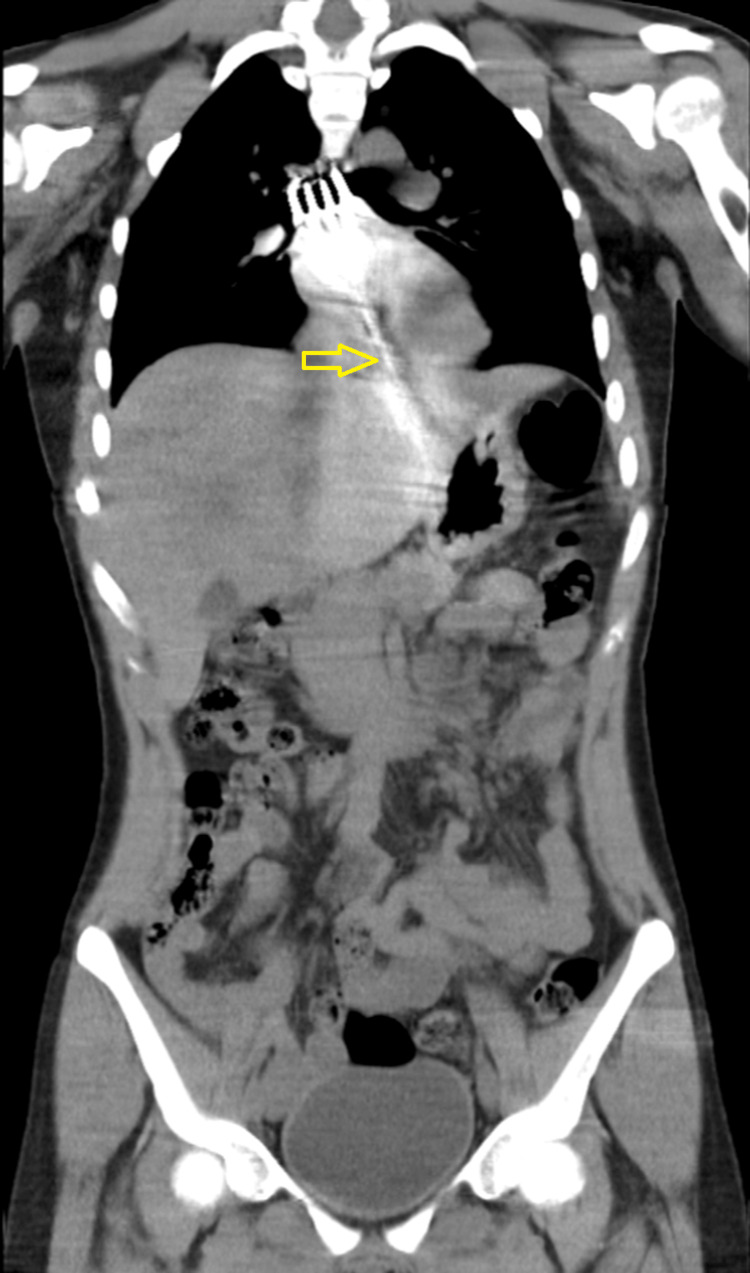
Abdominal CT confirming fork position without signs of perforation or hemorrhage. The gastroesophageal junction is marked by the yellow arrow.
